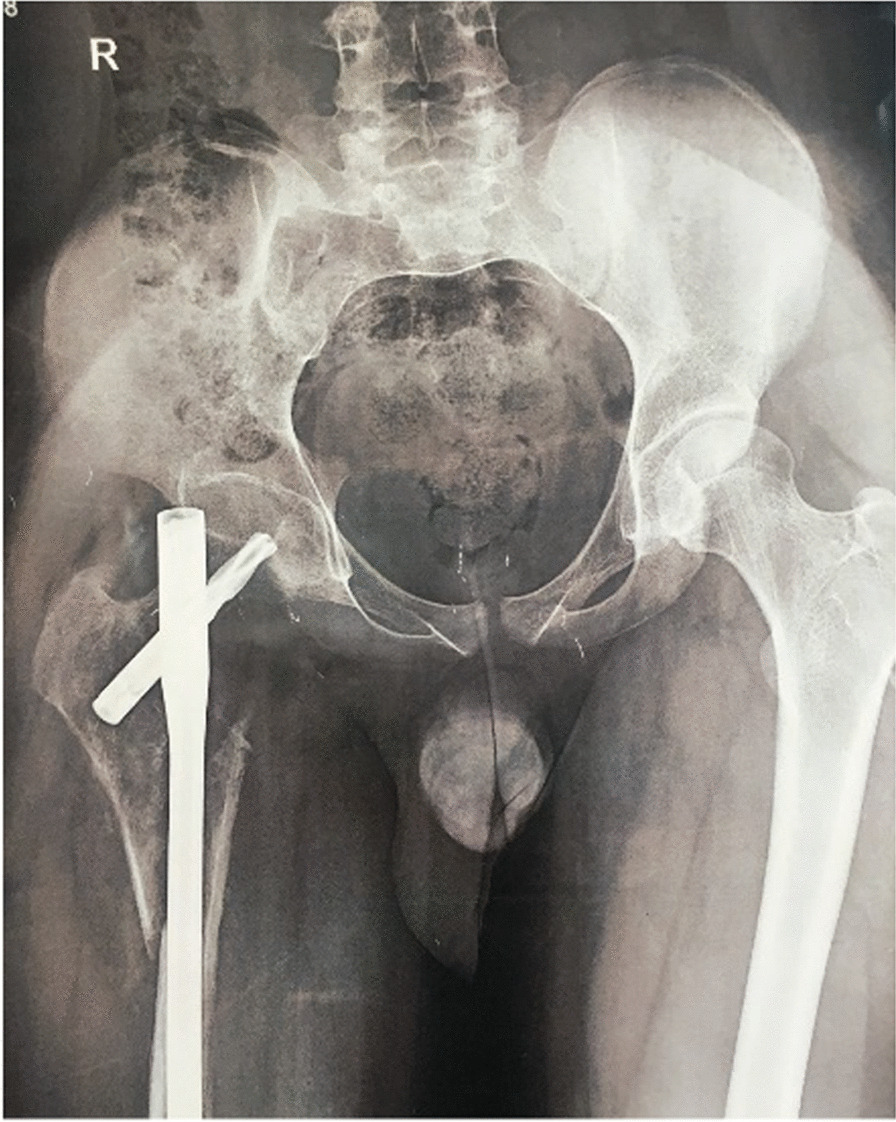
X-ray image showing the extensive lysis of the right trochanteric region and the femoral neck
